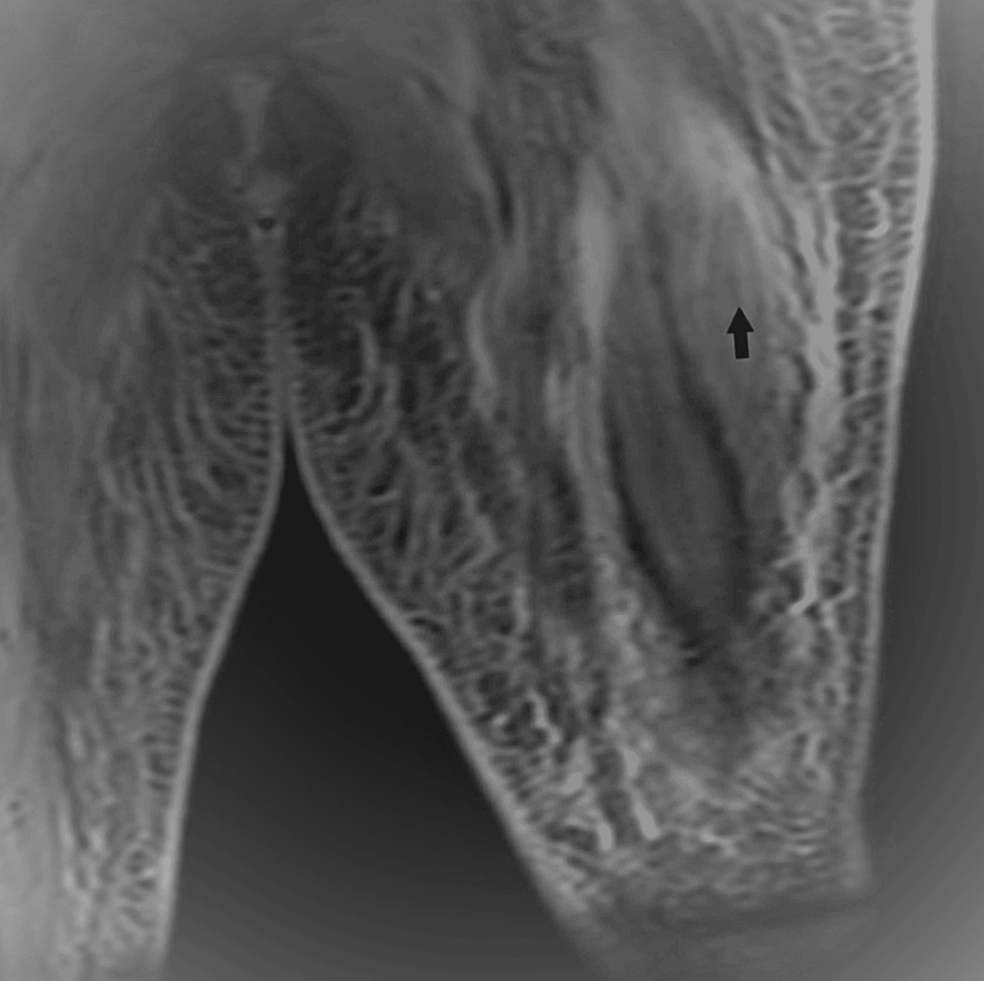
MRI left thigh STIR sequence MRI, magnetic resonance imaging; STIR, short tau inversion recovery.
